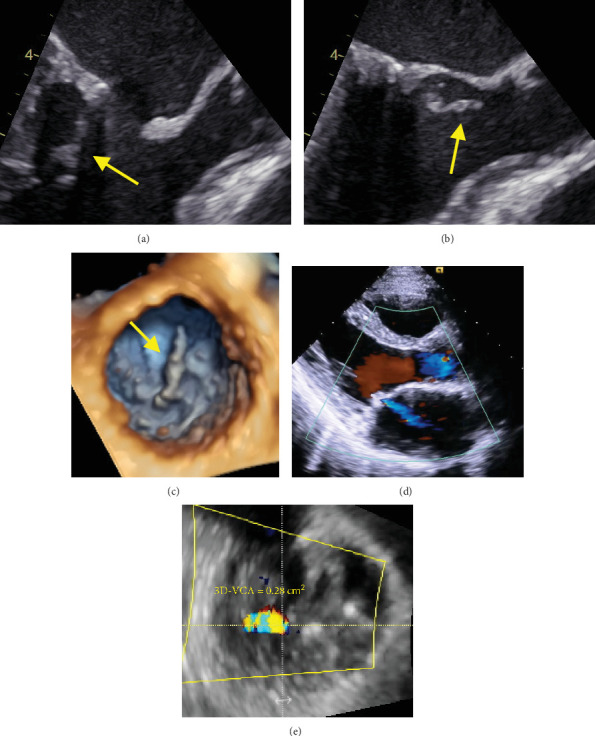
Echocardiographic images prior to patient discharge. Two-dimensional (2D) TEE long-axis zoomed view (120°) at late diastole (a) and mid-systole (b) and three-dimensional (3D) TEE en face view from the left ventricle (c) demonstrate a mobile structure attached to the posterior mitral leaflet (*yellow arrows*). 2D TTE color Doppler (d) and 3D TEE multiplanar reconstruction imaging (e) at mid-systole demonstrate central mild MR without leaflet prolapse.
